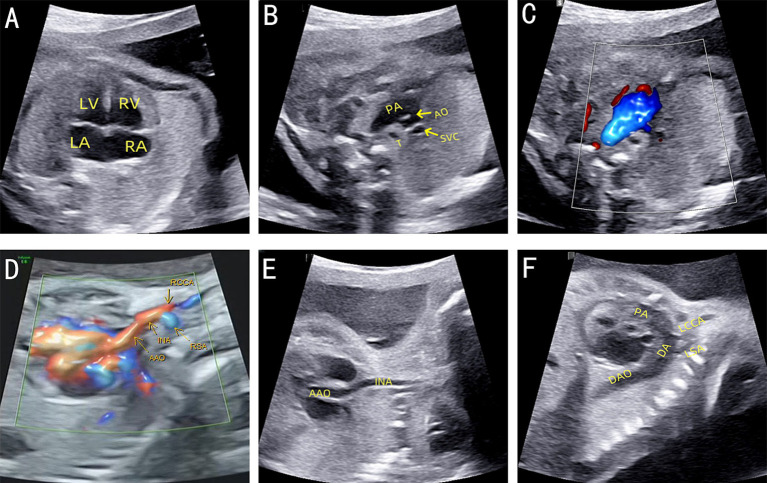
IAA Type C Prenatal Ultrasound. (A) The left and right heart proportions are basically symmetrical. (B,C) The pulmonary artery is significantly larger than the aorta, and the internal diameter of the aorta is slightly smaller than that of the superior vena cava. (D,E) The aortic arch continues only as the innominate artery. (F) The left common carotid artery and left subclavian artery connect to the descending aorta via the arterial duct.
